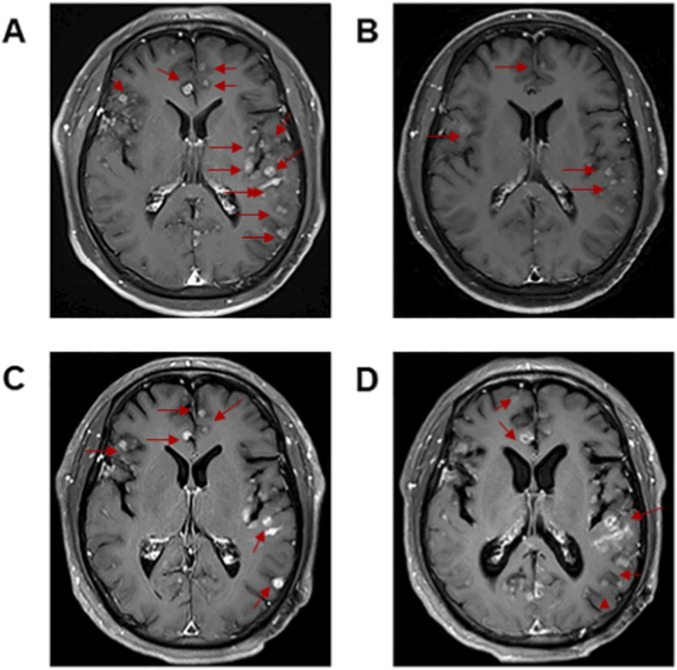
(A) Magnetic resonance imaging (MRI) scan of the brain before osimertinib therapy. (B) MRI scan of the brain after 15 days of osimertinib therapy. (C) MRI scan of the brain after 3.5 months of osimertinib therapy. (D) MRI scan of the brain after whole brain radiotherapy.
